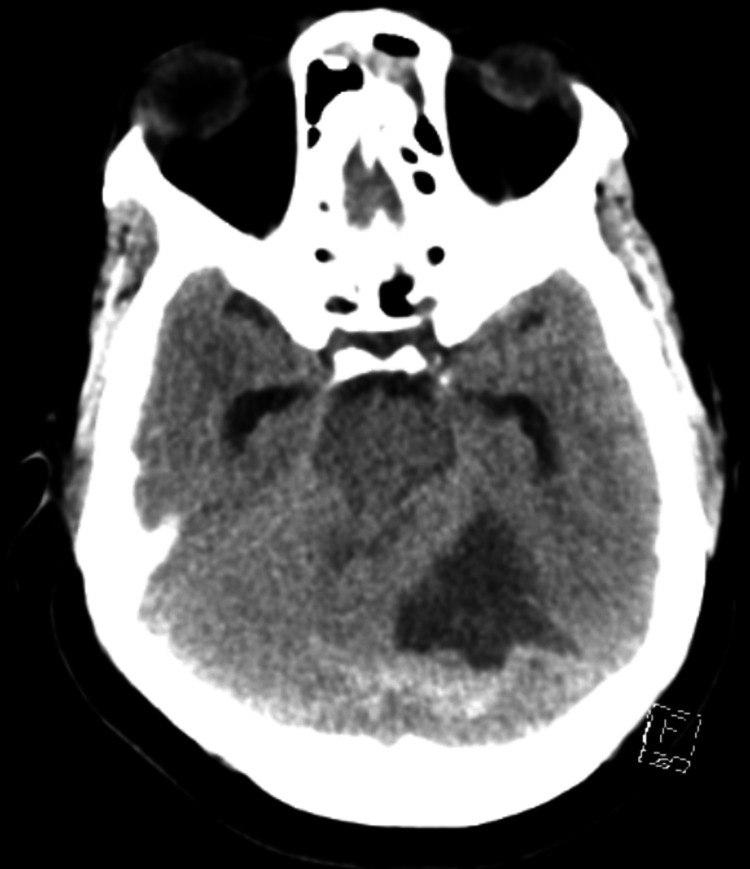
Axial View of Head CT Imaging showing obstructive hydrocephalus with effacement of the fourth ventricle and dilated lateral and third ventricles.
